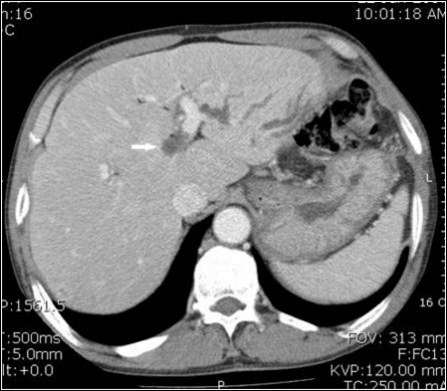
CT. The dilated left IHBDs are seen but otherwise it is normal. The arrow indicates a suspicious cyst on the review.
